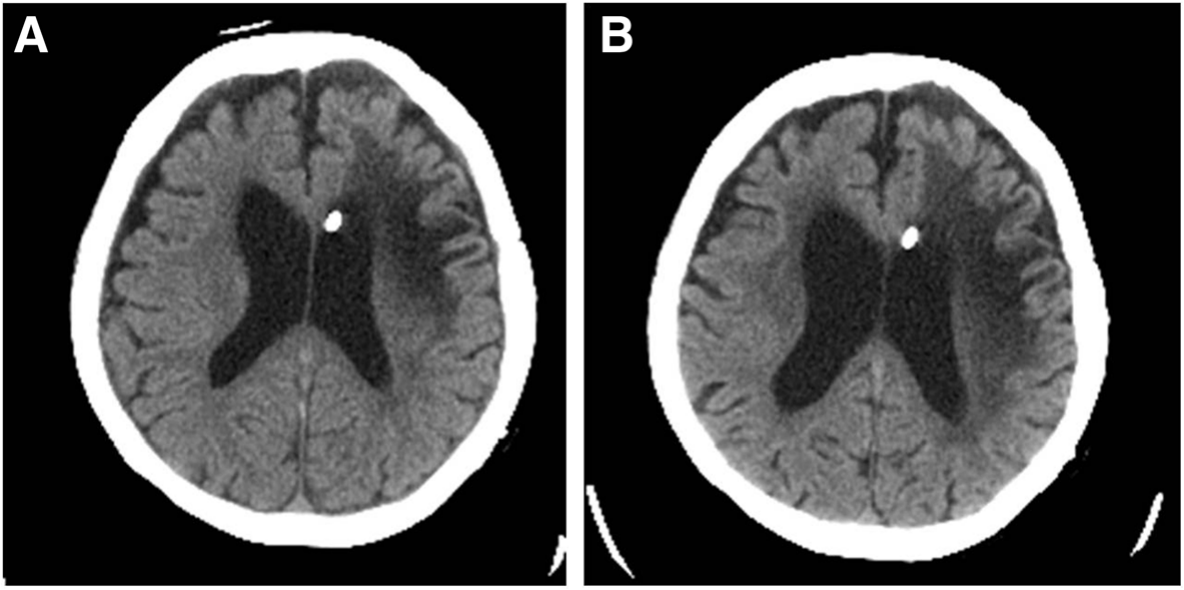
Brain CT showed that the ventricle was more dilated and the low-density region around the ventricle had expanded within 7 months (b) after the initial visit to our hospital (a)
